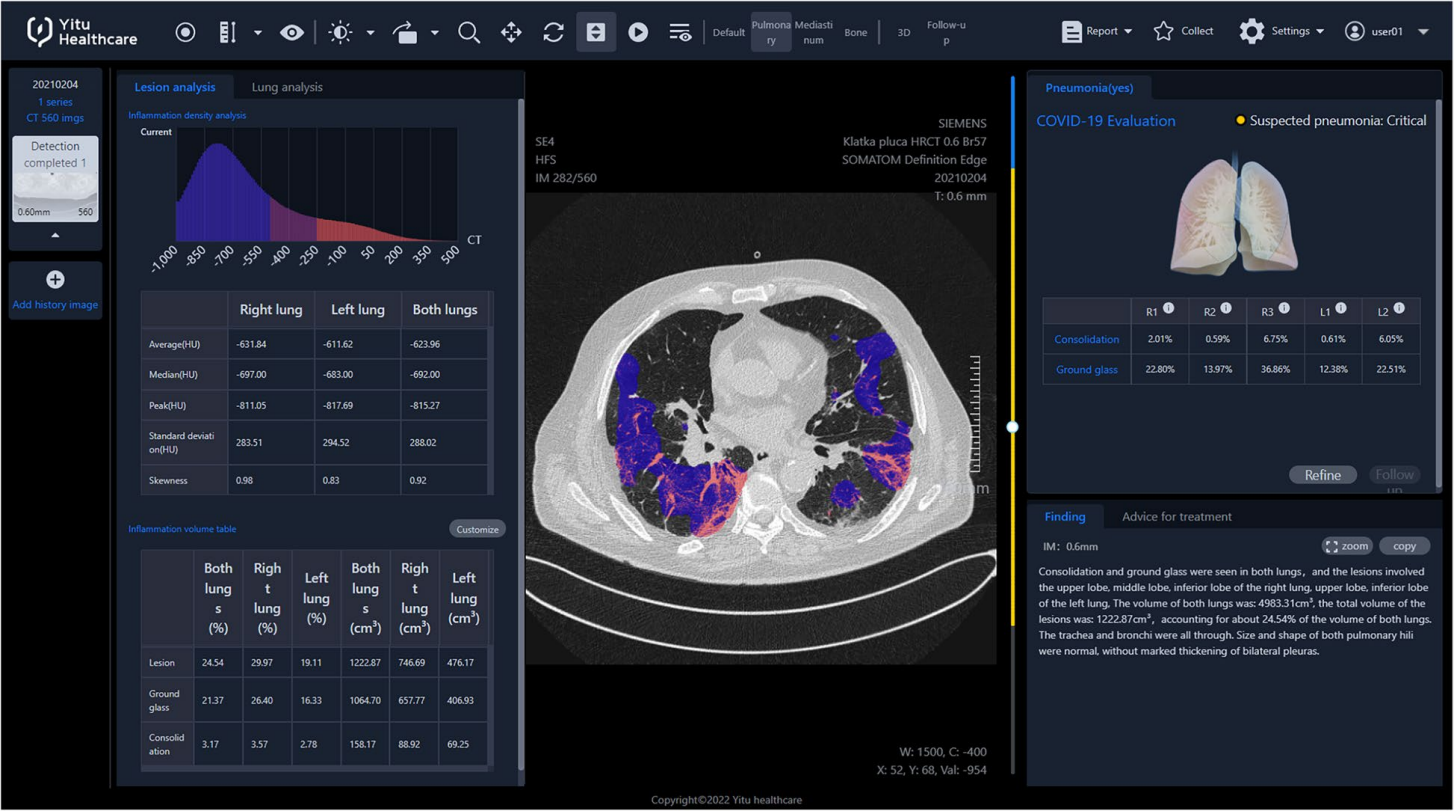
The final report from automatic analysis of HRCT by artificial intelligence technology
